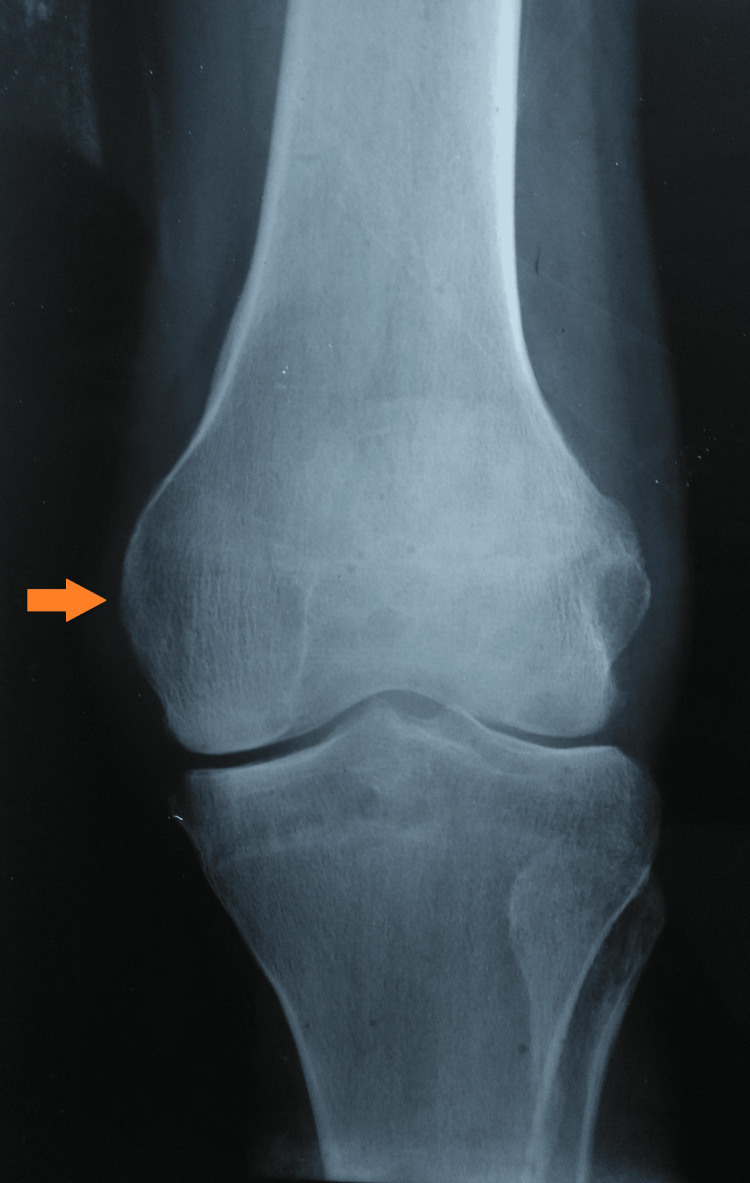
Plain radiograph of the left knee (AP view) showing no flaring-up of infection at the nine-month follow-up AP: anteroposterior
